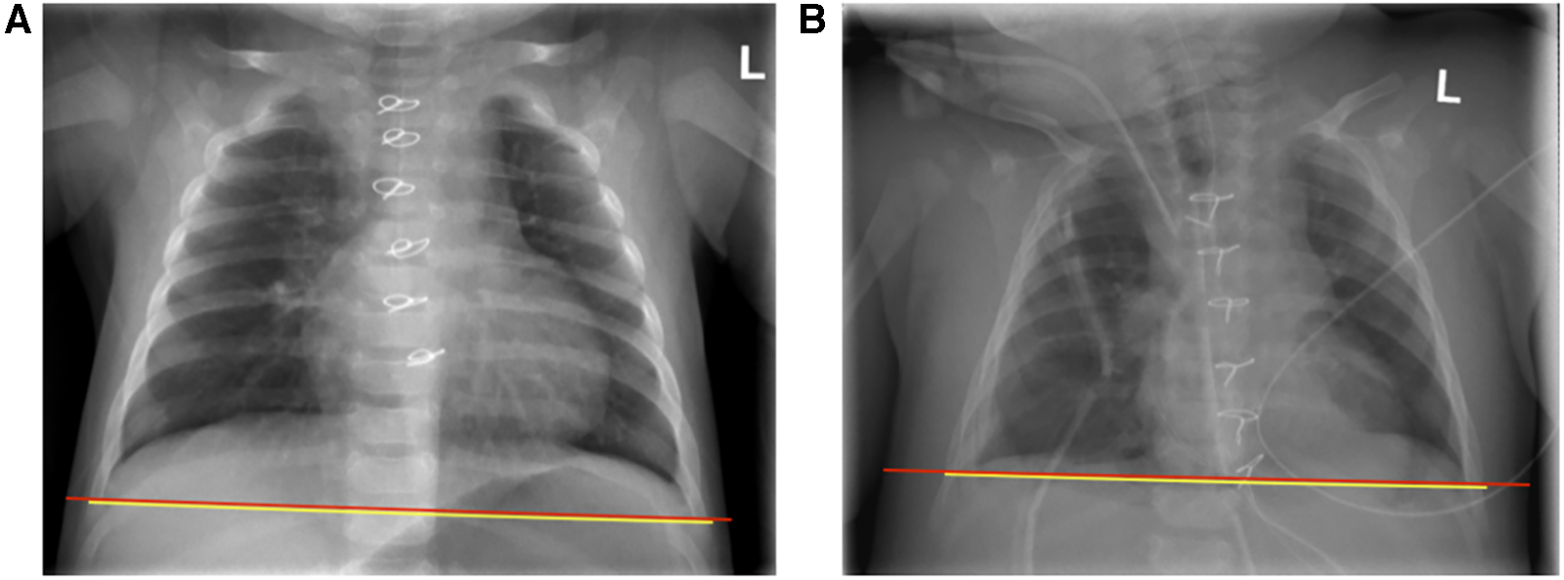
Example of chest x-ray analysis for assessment of subcutaneous-thoracic (S/T) ratio according to *sonntag* et al. (52) (A) Pre- and (B) postoperative x-rays of a patient with an S/T-ratio 16.8.
